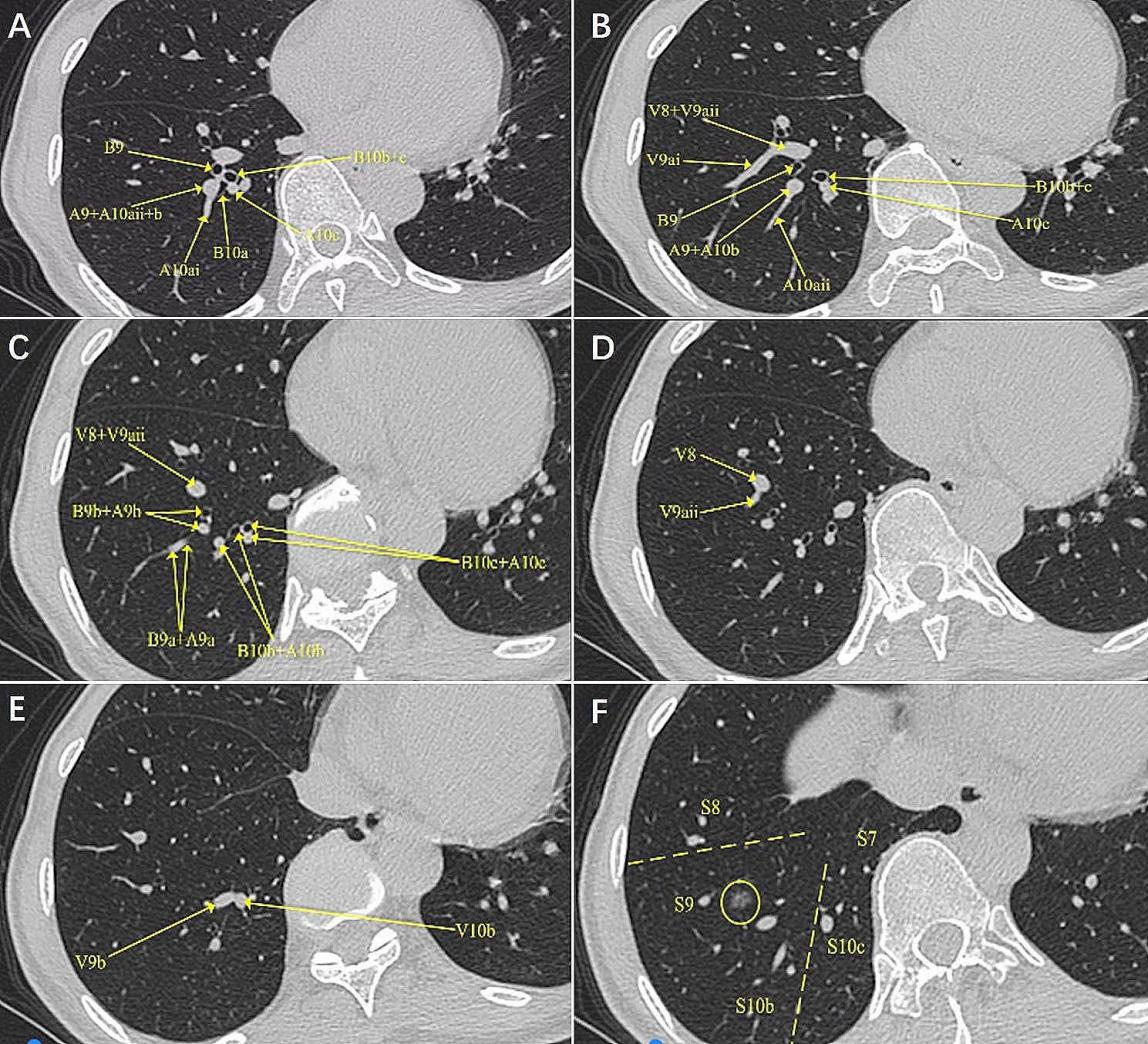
Identifying the subsegmental structures on preoperative HRCT. A, Identifying B9 and B10. B, Identifying V9ai and V9aii. C, Identifying A9a, A9b, and A10b + c. D, Identifying V8 and V9aii. E, Identifying V9b and V10b. F, Showing the extent the right S9 + 10 by bcombined subsegmentectomy
